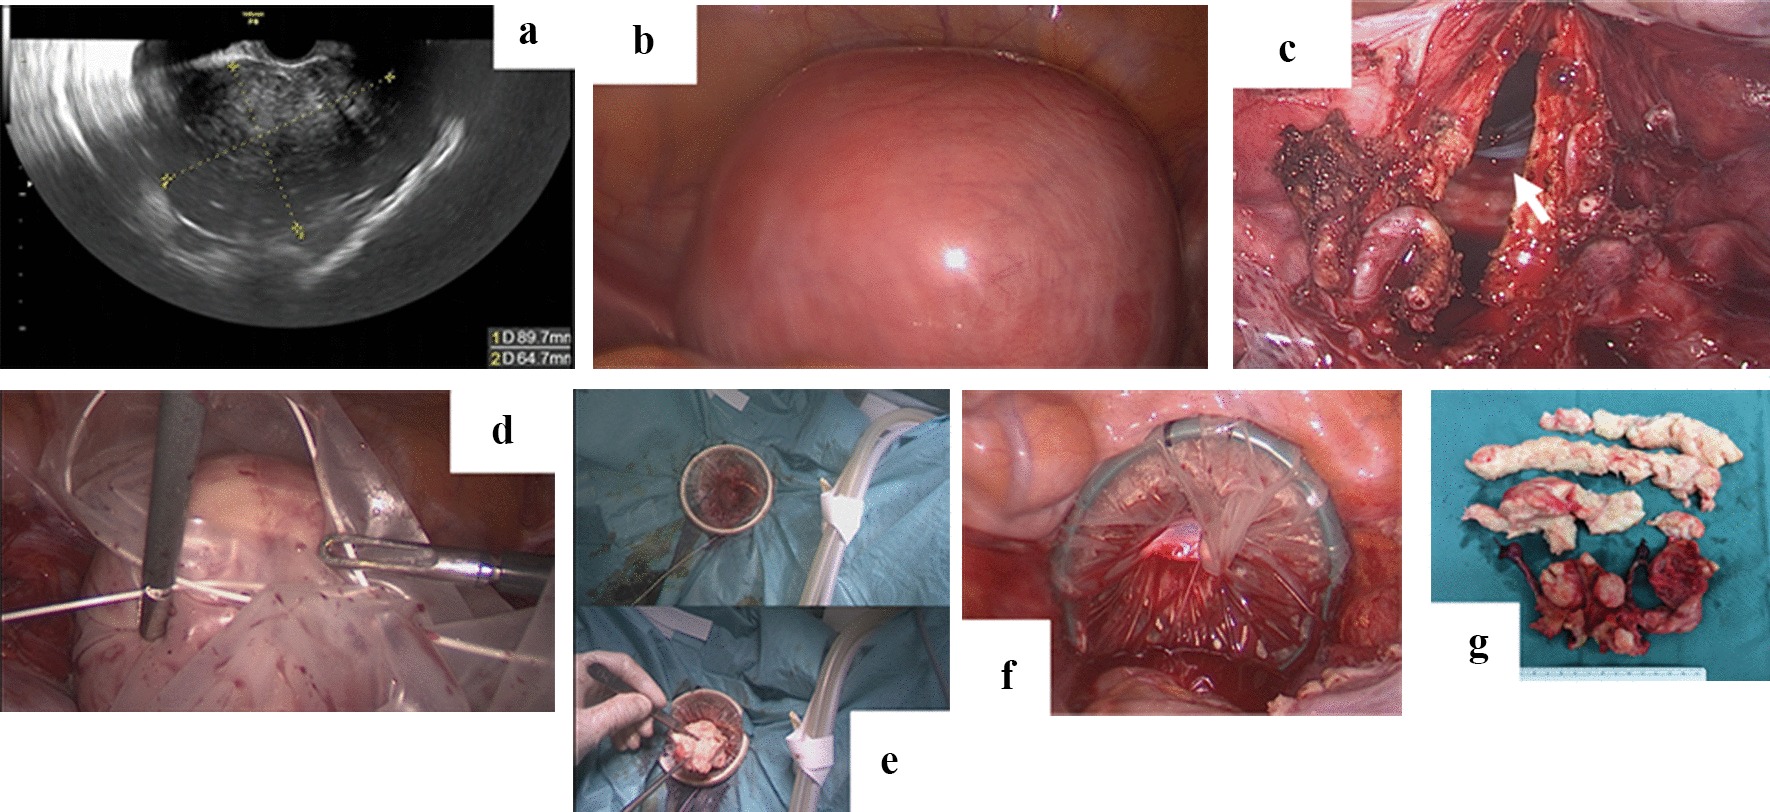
Surgical procedures of transvaginal morcellation of the uterus. This patient who underwent TLH and BS was a 47-year-old woman with multiple uterine leiomyomas. The largest leiomyoma was 8.4 cm in diameter, as detected by magnetic resonance imaging (MRI) during an outpatient examination. The size of the uterus was 90 × 65 mm, as detected by TVUS (a). She did not receive administration of GnRHa. She had a history of 0 gravidity and 0 parity, and her BMI was 20.9 kg/m^2^. The operation time was 134 minutes, and the blood loss volume was 75 ml. Four trocars were placed at the incision sites. The total weight of the uterus and bilateral tubes was 317.8 g. b Gross appearance of the uterus at the start of TLH. c Circumferential colpotomy using a cylinder-shaped vaginal pipe (arrow). d Collected uterus and tubes in 1200-ml MemoBag™. e, f Visualization of the vaginal wound with the Small Alexis^®^ Wound Protector/Retractor and morcellated uterus (e inside, f outside). g Gross appearance of the uterus and tubes removed during surgery
